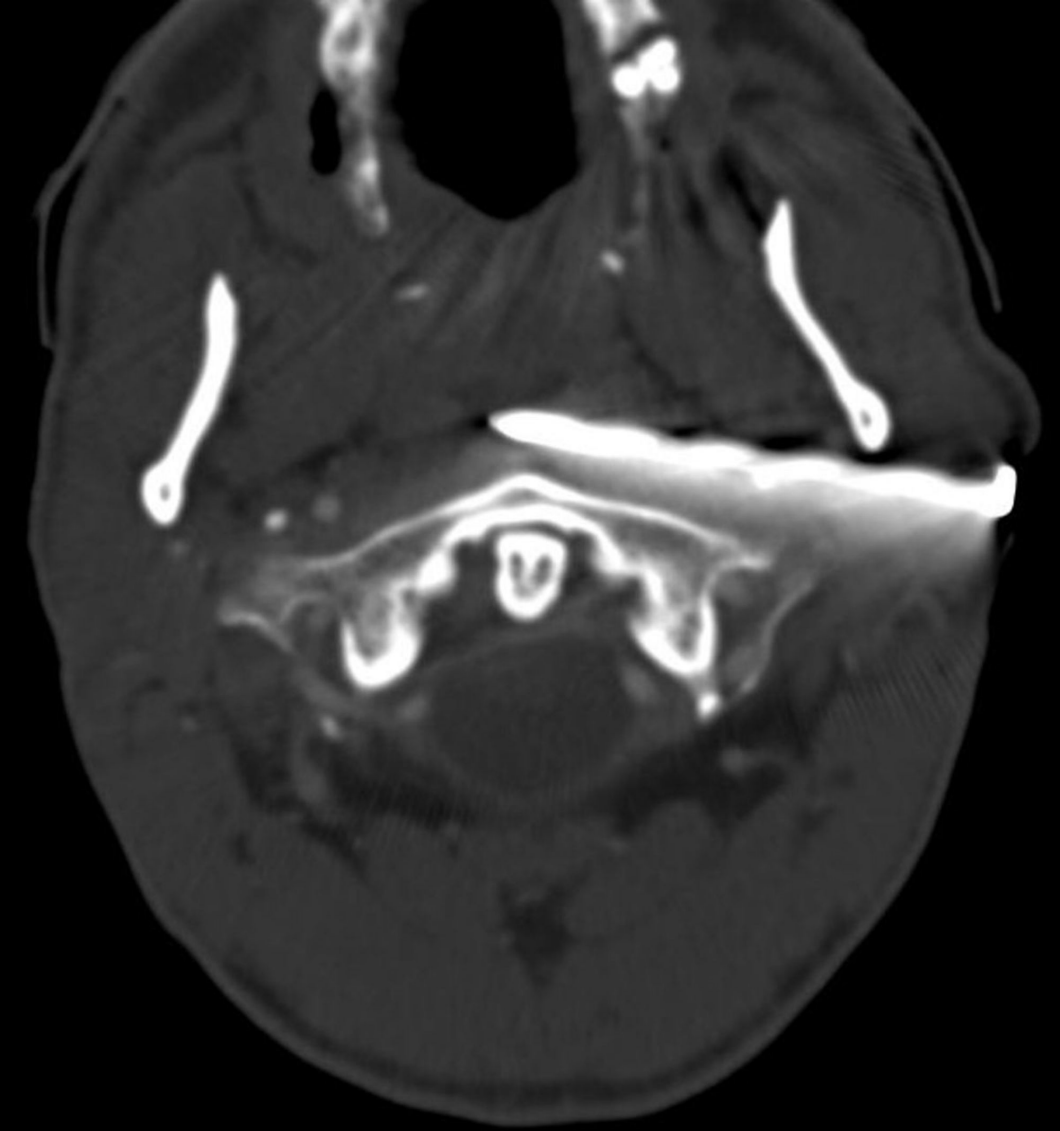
Computed tomography angiography demonstrating nail in the retromandibular region traversing in close proximity to the cervical left internal carotid artery (obscured by metallic artifact from the nail)
